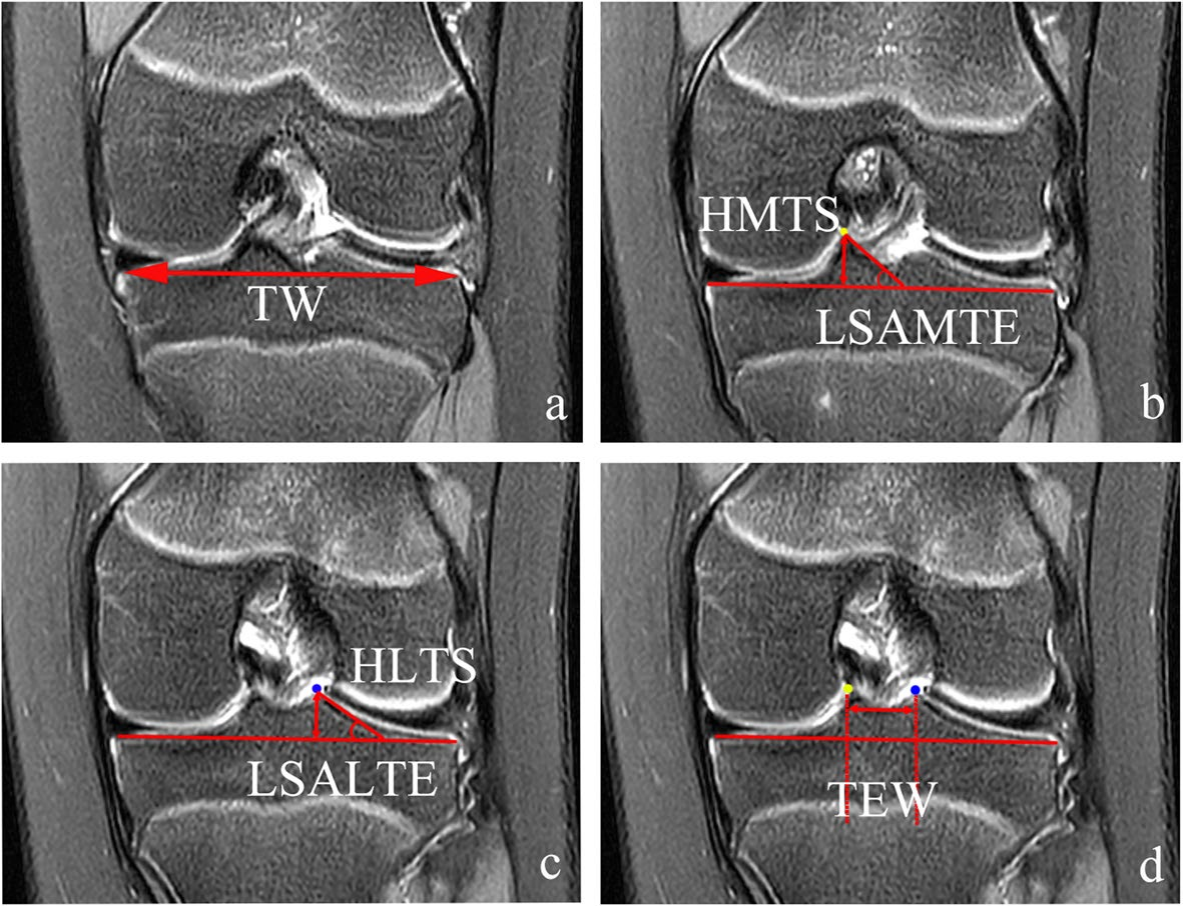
MRI parameters measured for evaluation of tibial plateau morphology. Coronal proton density-weighted MR images showing: a TW, maximum tibial plateau width (double-headed arrow). b On the slice showing the peak of the medial tibial eminence (yellow point), HMTS was the distance from the tip of the medial tibial spine to the imaginary tibial joint line (double-headed arrow), and LSAMTE was the angle between the line drawn along the lateral slope of the medial tibial spine and the imaginary tibial joint line. c On the slice showing the peak of the lateral tibial eminence (blue point), HLTS was the distance from the tip of the lateral tibial spine to the imaginary tibial joint line (double-headed arrow), and LSALTE was the angle between the line drawn along the lateral slope of the lateral tibial spine and the imaginary tibial joint line. d TEW was the distance between the peak of the lateral and the medial tibial eminence (double-headed arrow). Note that the positions of the yellow point and the blue point are different on the coronal images
